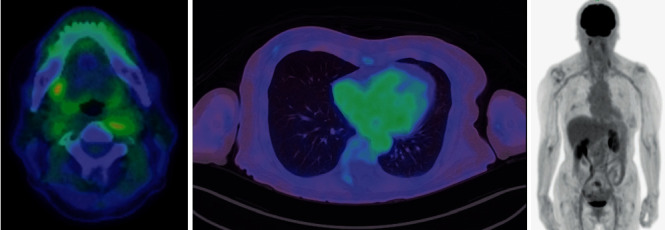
At 6 months after treatment, PET-CT no longer showed increased glucose uptake by the lesions.
